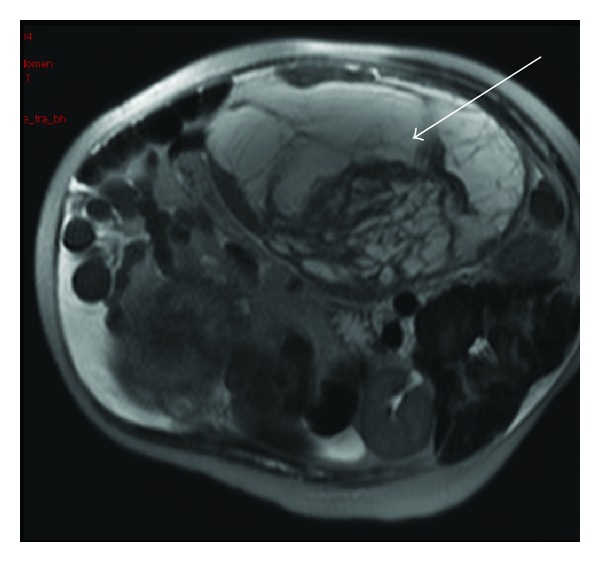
MRI abdomen, arrow indicating complex ovarian mass.
